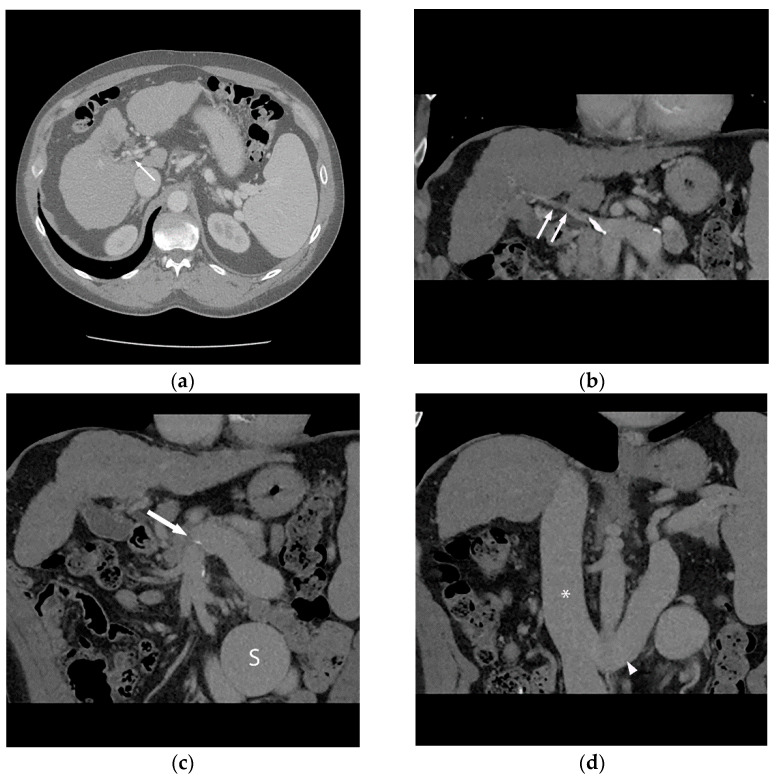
56-year-old male with history of alcohol-related cirrhosis and portopulmonary syndrome. His baseline values included MELD-Na 19 (Tbili 4.0, INR 2.0, Cr 1.0, Na 137). He had imaging evidence of non-occlusive main portal vein thrombosis. Axial (a) and coronal (b) contrast-enhanced CT shows a diminutive portal vein (arrow). Coronal contrast-enhanced CT (c,d) demonstrates this extended to the portal venous confluence (arrow), with partially visualized component of a large inferior mesenteric vein to gonadal vein portosystemic shunt (S), which drained via the renal vein (arrowhead) to the inferior vena cava (*).
